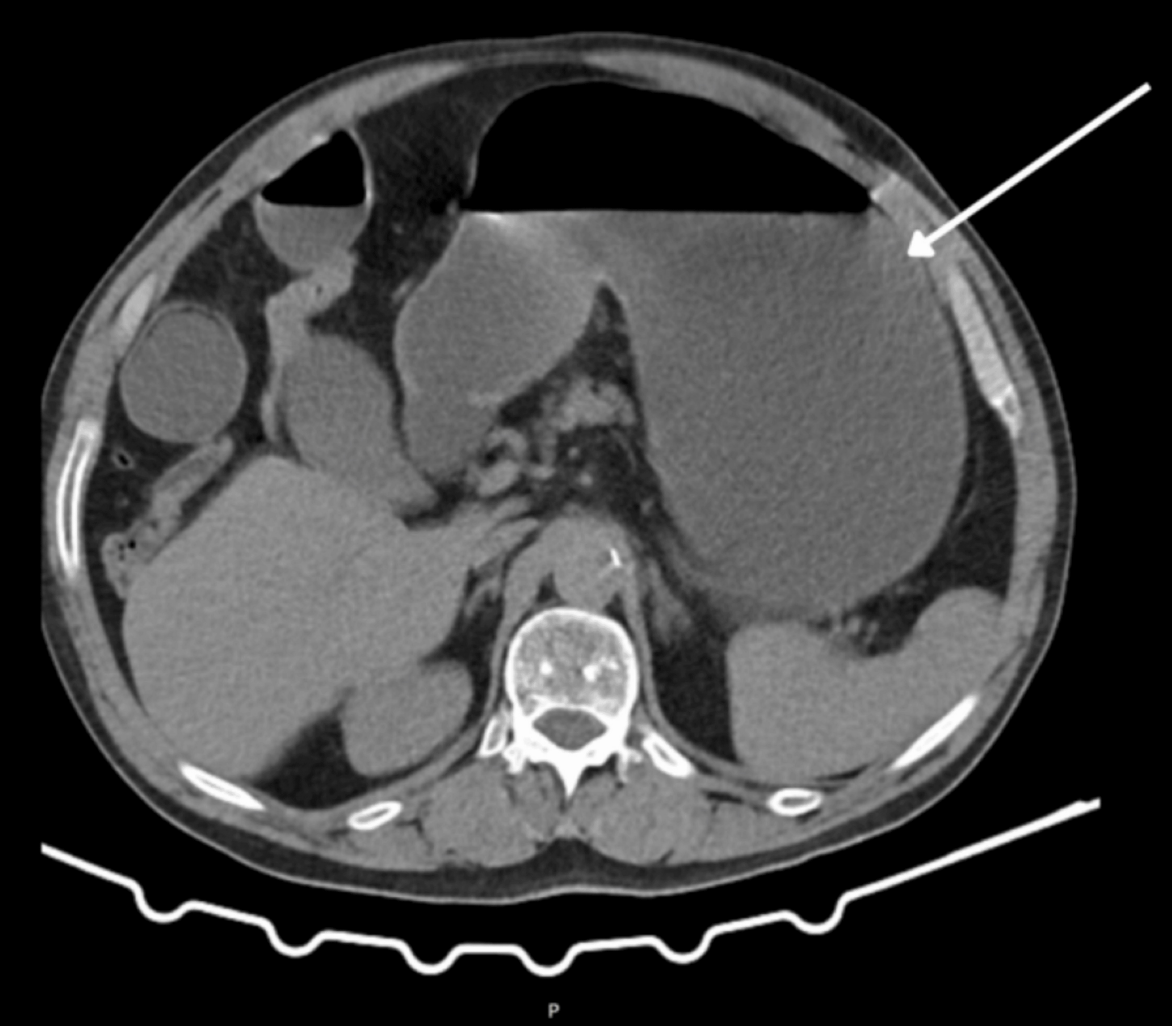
CT scan image - axial section This CT Scan shows gastric distention (arrow).
